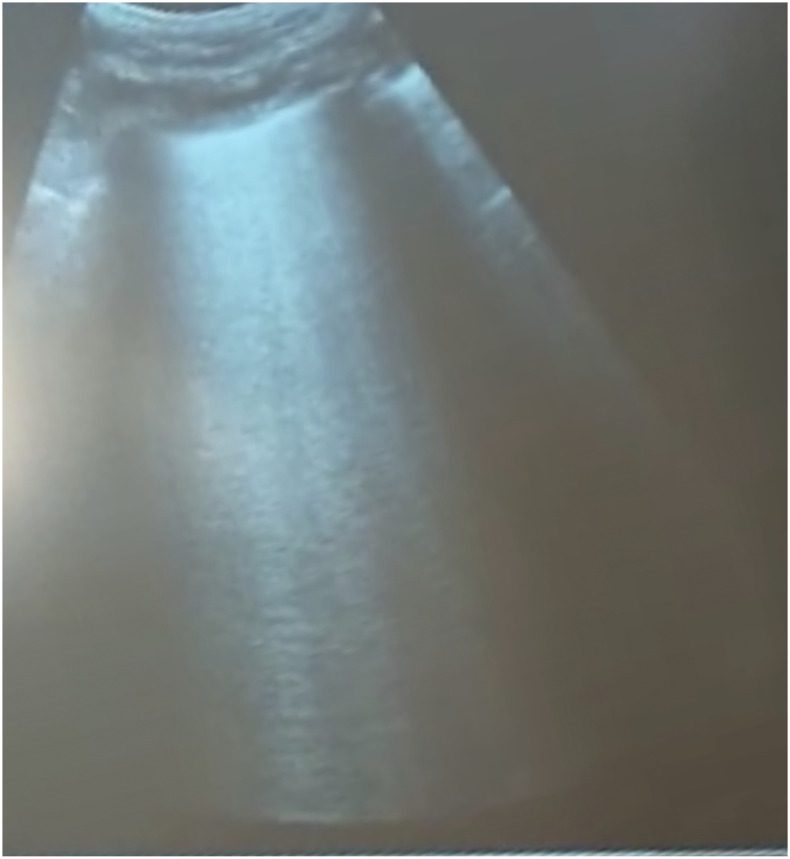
B lines were followed on point-of-care ultrasound documenting the worsening acute respiratory distress syndrome.
